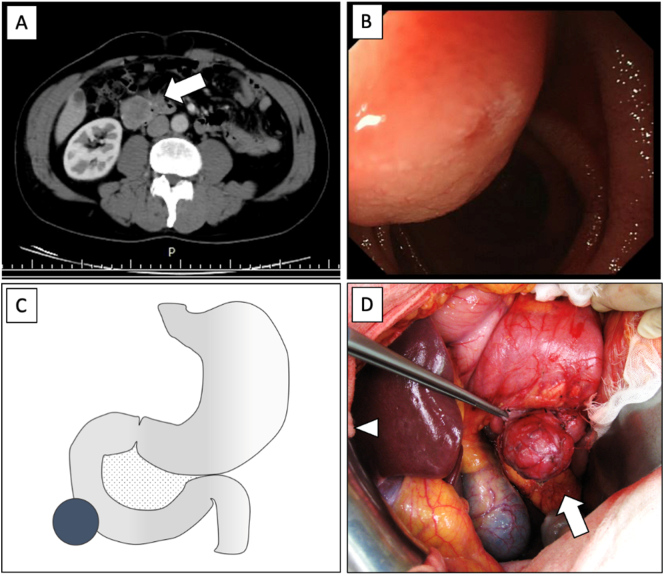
Tumour location and findings in patient two. (A) Abdominal enhanced CT scan shows a 3-cm-sized heterogenous enhanced tumour in the anterior wall of the 2nd portion of the duodenum. Arrow, tumour. (B) Endoscopic findings demonstrate an SMT in the 2nd portion of the duodenum. (C) Scheme for the site of the tumour. •, tumour. (D) No invasion to the adjacent organs is observed. Arrow, tumour; Arrowhead, cranial side of the body. Abbreviation: SMT, submucosal tumour.
